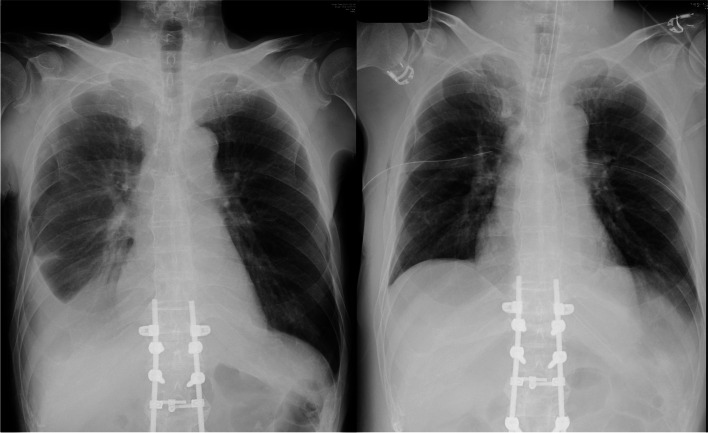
Chest radiography of pre-operation (left) and post-operation (right)
